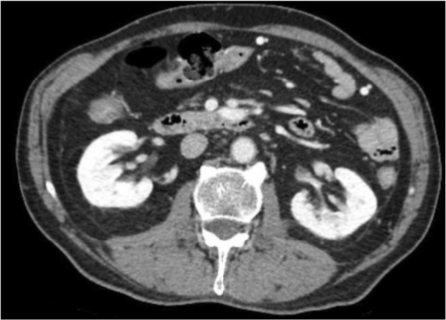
18 months after HIFU, CT shows the complete response at the level of metastatic lymphnodes
